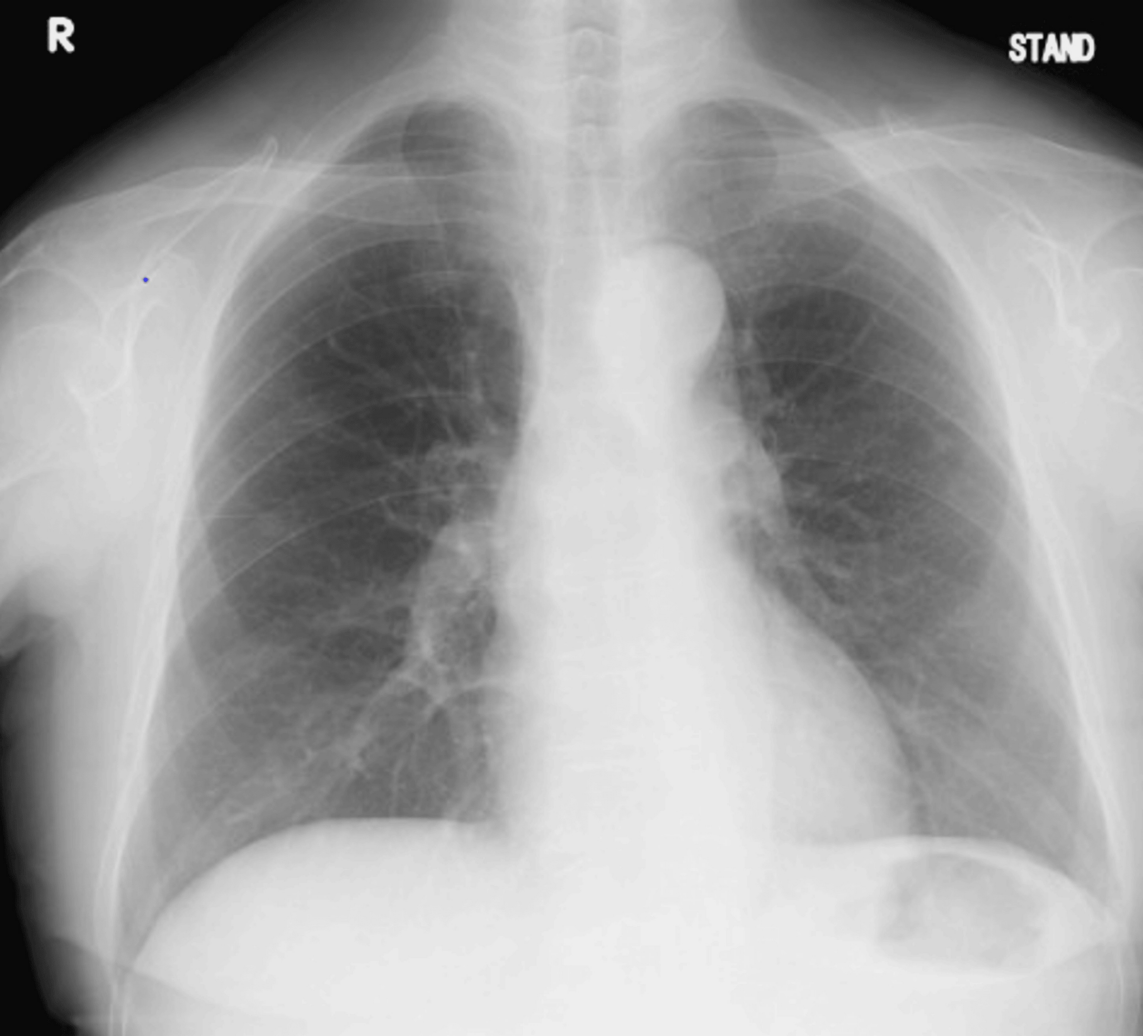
Radiography two months after onset of chylothorax There is no relapse of chylothorax.
